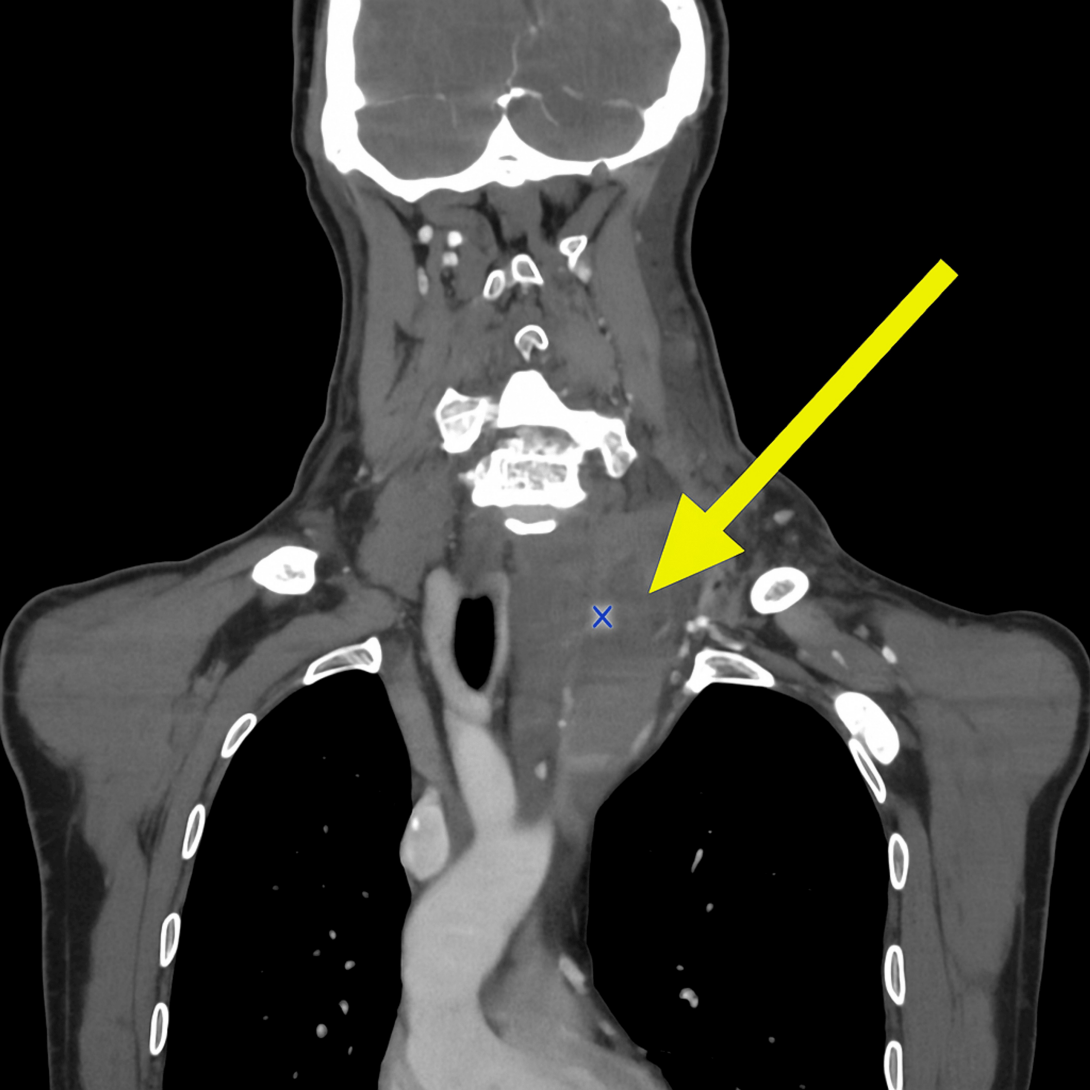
Contrast-enhanced CT scan of the neck (coronal view) demonstrating a well-defined, soft-tissue mass (yellow arrow) located in the lower cervical region. Scanned locally within Pilgrim Hospital, United Lincolnshire Teaching Hospitals NHS Trust
